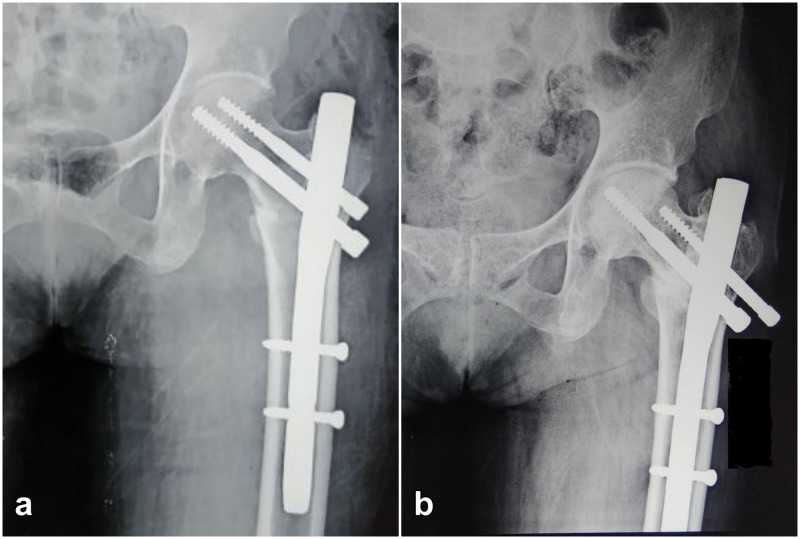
Radiograph of 65-year-old female patient with screw back-out. (a) Radiograph taken on day 1 post-surgery. (b) Radiograph taken six months post-surgery showing reverse-Z effect and fracture union.
